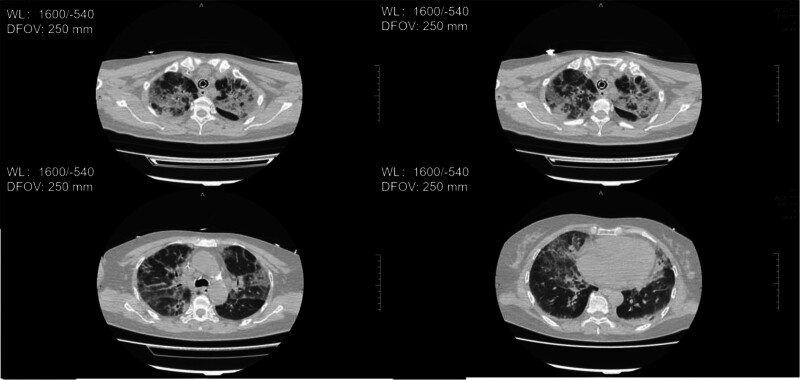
Chest CT on September 13, 2023, showed diffuse bilateral patchy and strip-like high-density opacities, indicating persistent but evolving pulmonary infiltrates. CT = computed tomography.
